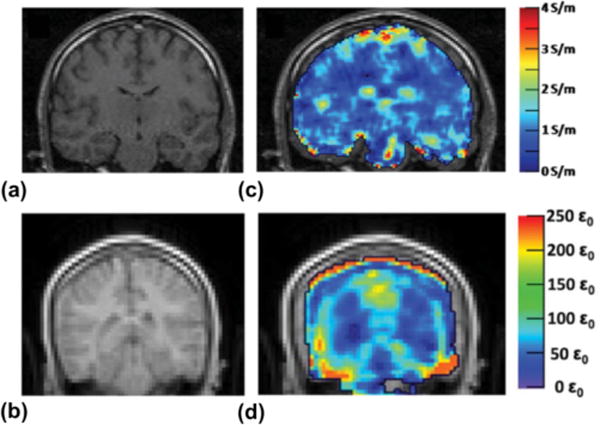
Results of reconstructed EPs of healthy human brain tissue *in vivo*. (a) and (b) Magnitude of MRI data for reconstruction of EPs in (c) conductivity and (d) permittivity, respectively. EPs were reconstructed using equation (10), magnitude *B*_1_^+^ and estimated phase based on TPA. Reproduced with permission from Voigt, Katscher and Doessel. Magn Reson Med 2011; 66:456–466.
